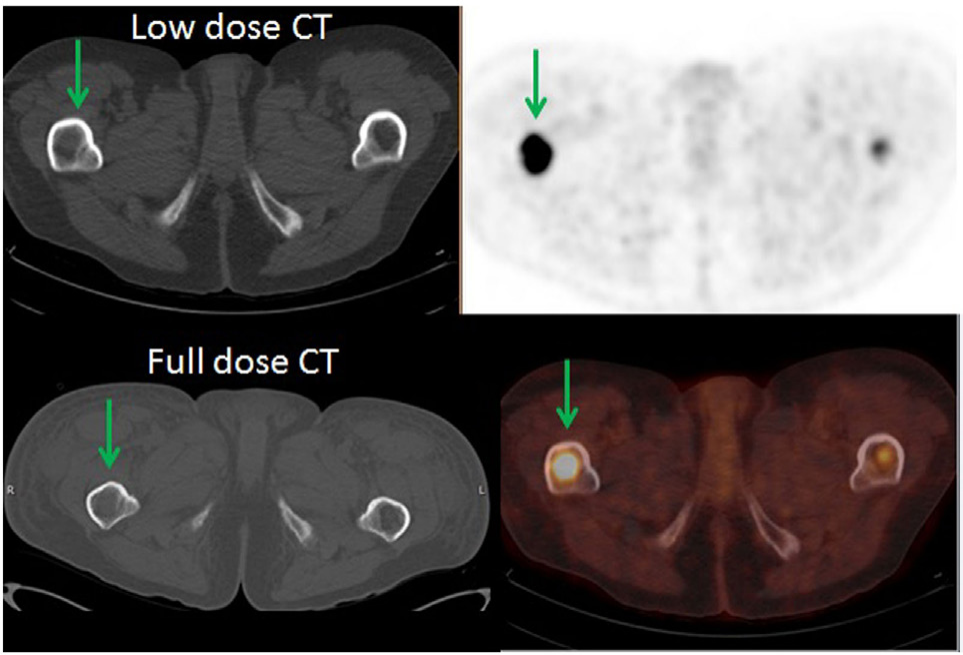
A 53-year-old male diagnosed with diffuse large B-cell lymphoma with multiple FDG-avid lesions above and below the diaphragm. There were PET-positive CT-negative femoral lesions (green arrows). Patient had a full dose CT which was negative for SM. There was no change in staging but XRT and BMA were offered.
